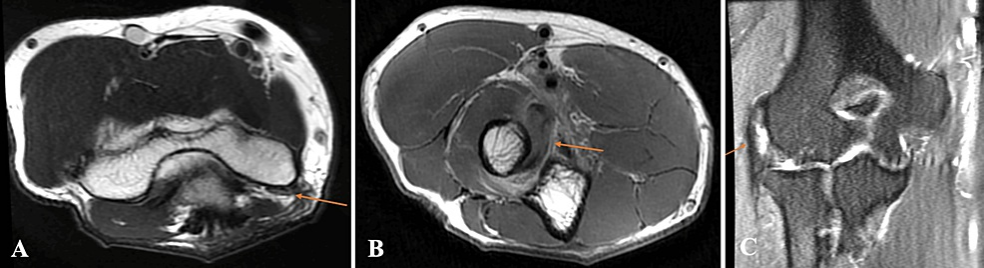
Preoperative magnetic resonance imaging without contrast of the right elbow. Coronal T1 view (A) with an arrow identifying inflammation of the ulnar nerve in the cubital tunnel. Axial T1 view (B) with an arrow identifying complete disruption of distal biceps tendon proximal to radial tuberosity. Coronal T2 view (C) with an arrow identifying severe partial tear of the common extensor tendon at the level of the lateral epicondyle.
